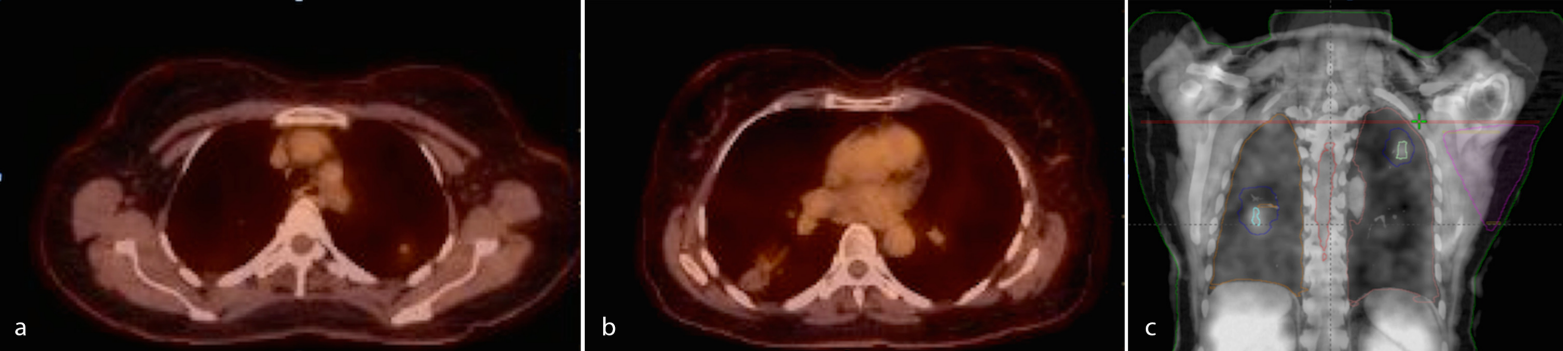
Positron emission tomography-CT scan axial (a, b) and coronal (c) images show fludeoxyglucose-avid lesions in the left upper lobe (maximum standardized uptake value of 1.6) and right lower lobe (maximum standardized uptake value of 2.0).
